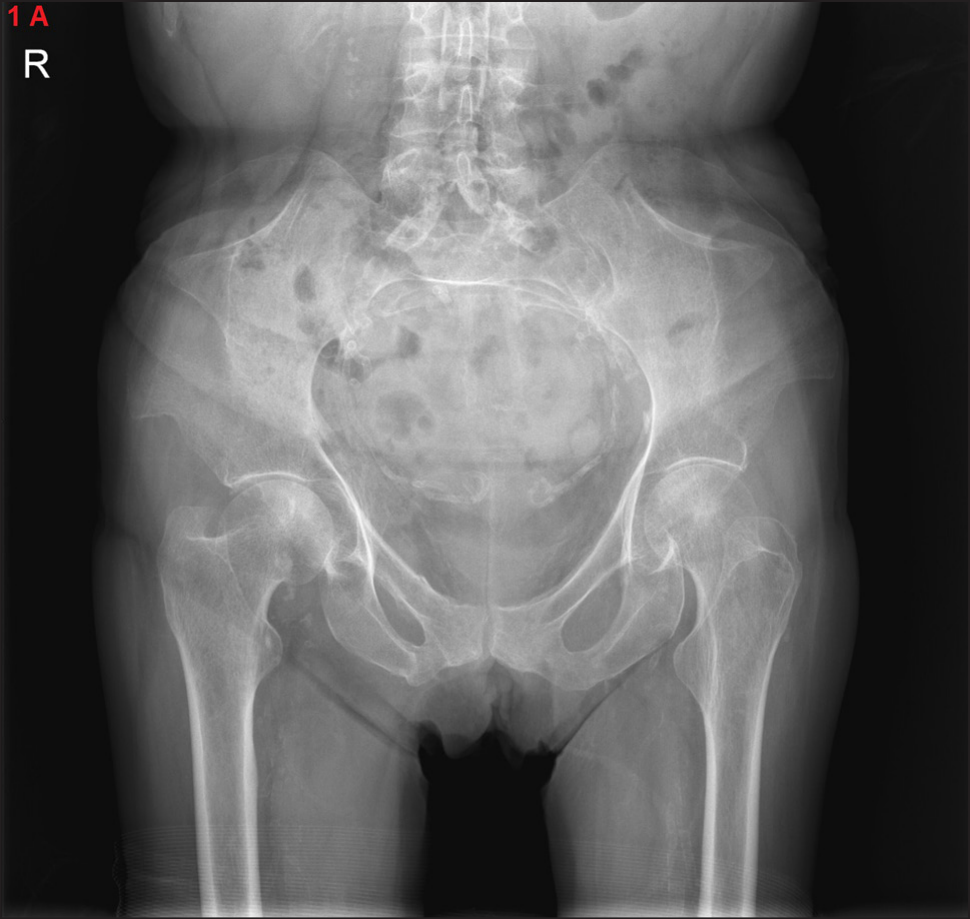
Anteroposterior radiograph of the pelvis shows the presence of bilateral triangular osseous outgrowth from the iliac bones.
